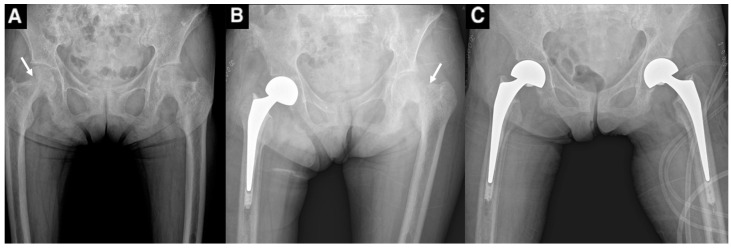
(A) An anteroposterior radiograph of the pelvis showing osteoporotic bone with a spontaneous femoral neck fracture on the right side (narrow arrow). (B) Cemented hemiarthroplasty was performed, but the patient developed a spontaneous fracture on the contralateral side after 7 days (narrow arrow). (C) A resultant X-ray showing cemented hemiarthroplasty on both sides.
